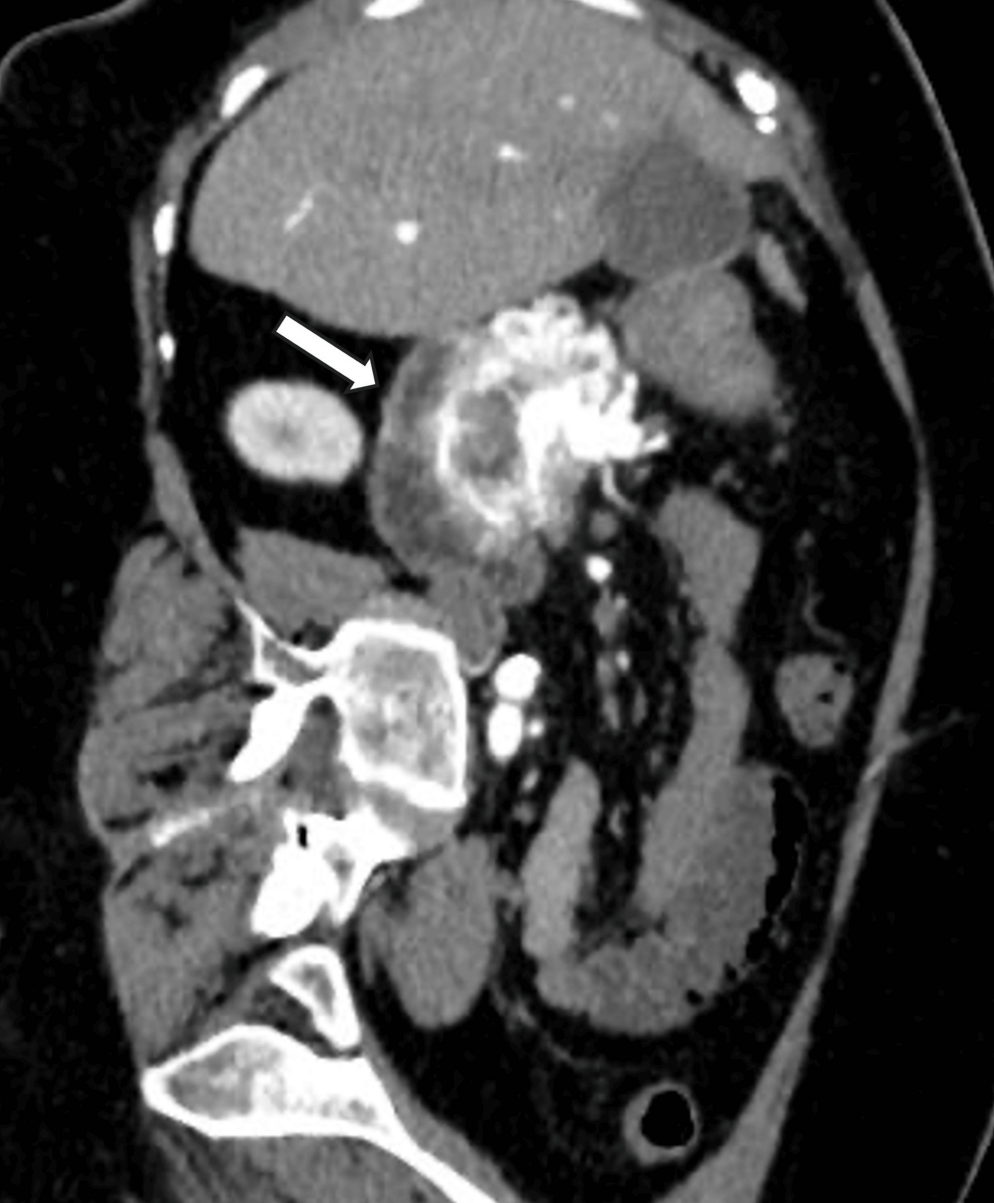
CT showing pancreatic head mass without duodenal invasion Contrast-enhanced CT of the abdomen, oblique reconstruction, shows a solid mass in the pancreatic head that contacts and displaces the first and second portions of the duodenum, without associated mural thickening, luminal narrowing, or obstruction to suggest direct invasion (arrow). CT: computed tomography
